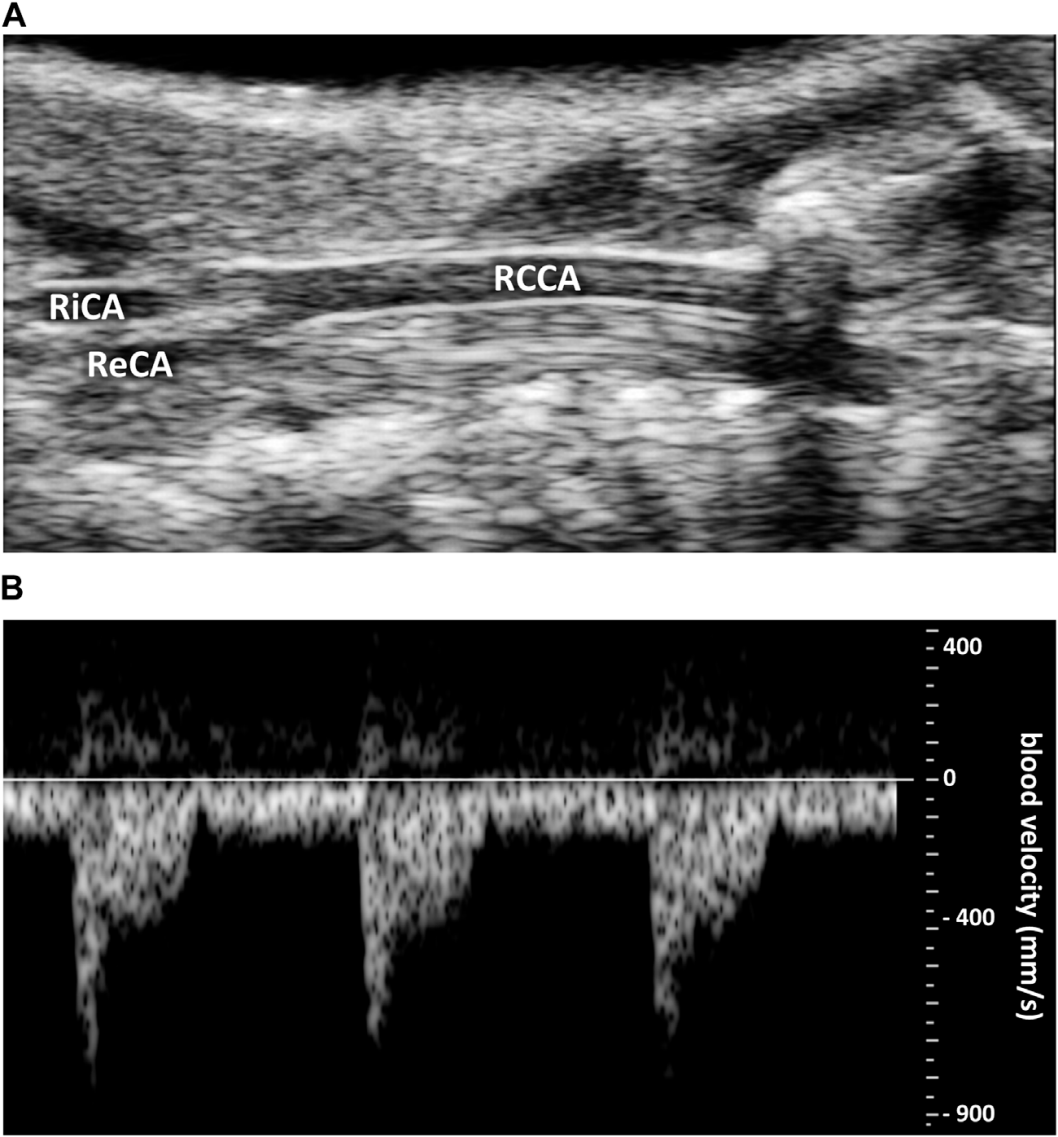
Representative original traces of carotid ultrasound imaging. (A) Right common coronary artery (RCCA) diameter. RiCA, right internal carotid artery. ReCA, right external carotid artery. (B) Blood velocity profile (in mm.s^−1^).
